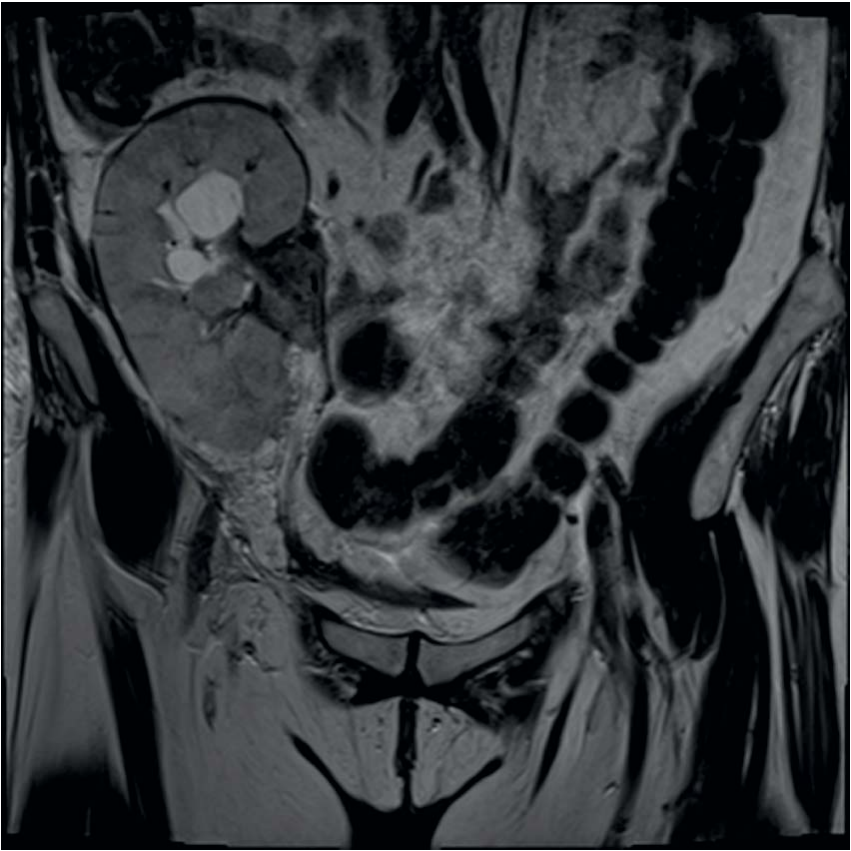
Magnetic resonance imaging, coronal T2-weighted image. Intermediate and low signal intensity mass is present in the renal hilum infiltrating the renal pelvis and surrounding normal vascular structures.
